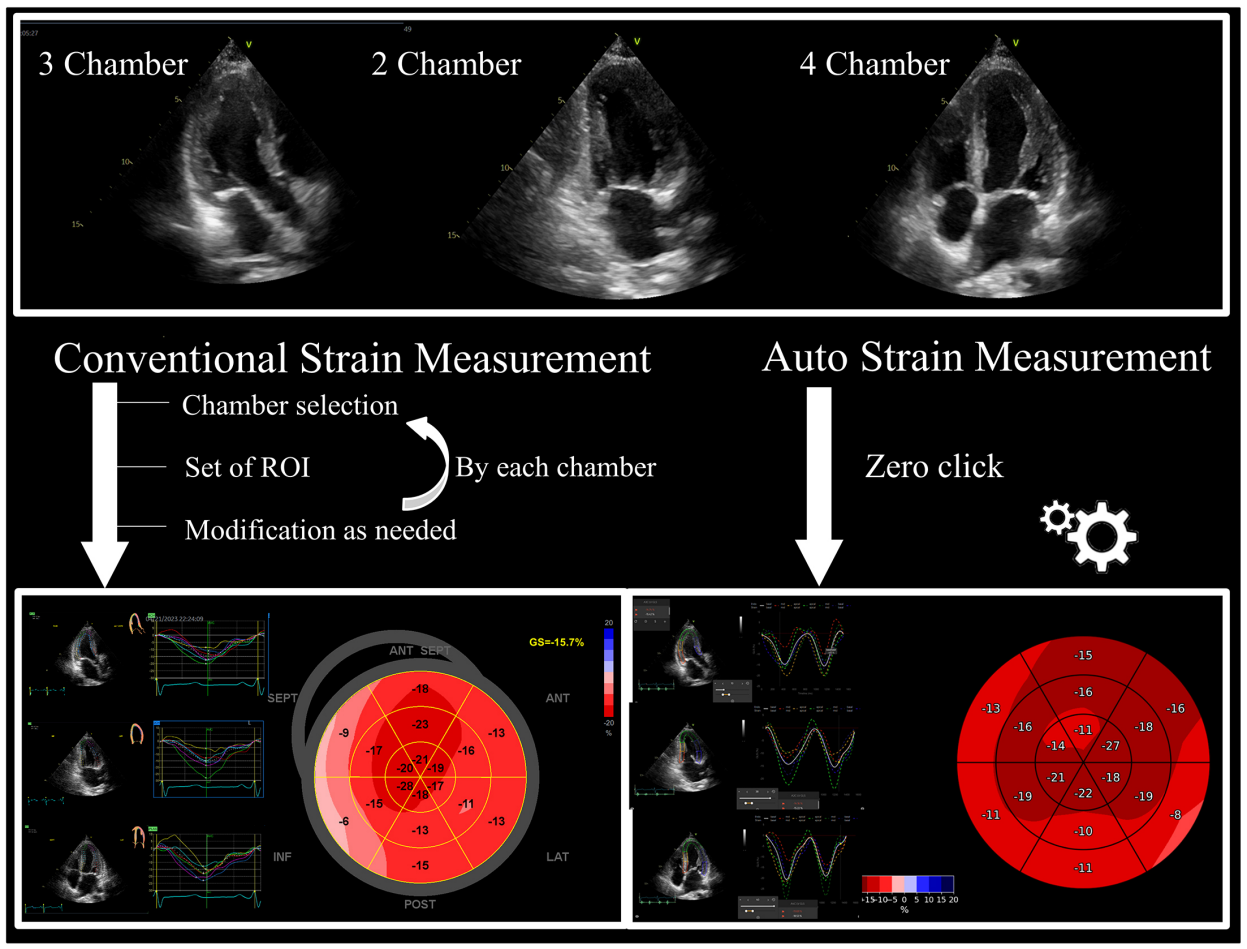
Comparison between conventional and automated strain measurements in echocardiography Conventional measurement involves manual steps like chamber selection and ROI modification (left). Automated measurement (right) requires no manual intervention and provides instant results. ROI: region of interest.
